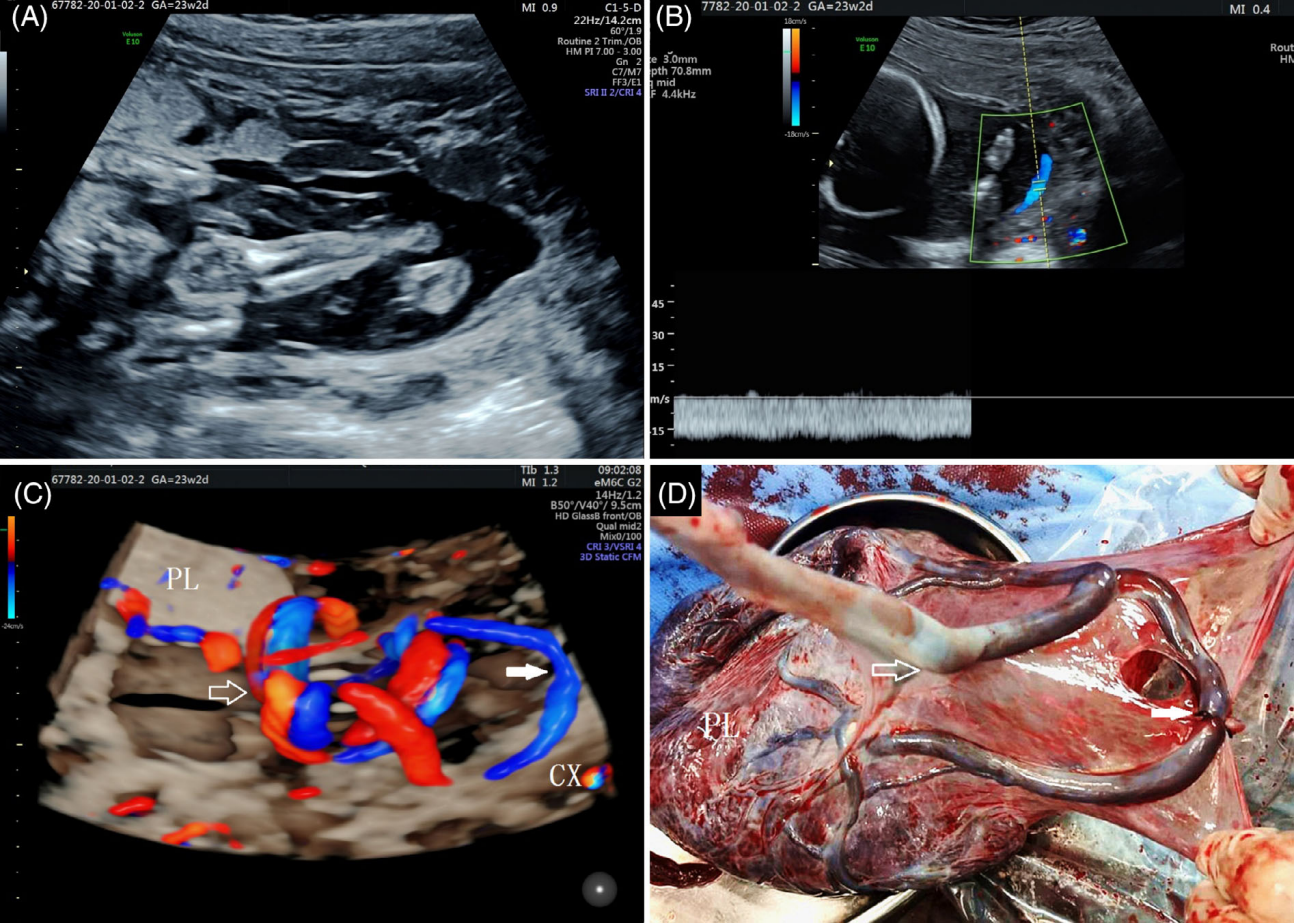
Two‐dimensional ultrasound showing tubular hypoechoic structure near the cervical os suggesting vessel previa (A). Color Doppler imaging demonstrating venous blood flow spectrum near the cervical os (B). Flow HD glass body of the umbilical cord showing sail‐like insertion and vessel previa through the cervix (C). PL, placenta; CX, cervix; hollow arrow, umbilical cord insertion point; solid arrow, sail‐like vessel. Postpartum image of placenta, umbilical cord, and umbilical vessels showing sail‐like insertion point (D), PL, placenta; hollow arrow, umbilical cord insertion point; solid arrow, sail‐like vessel.
